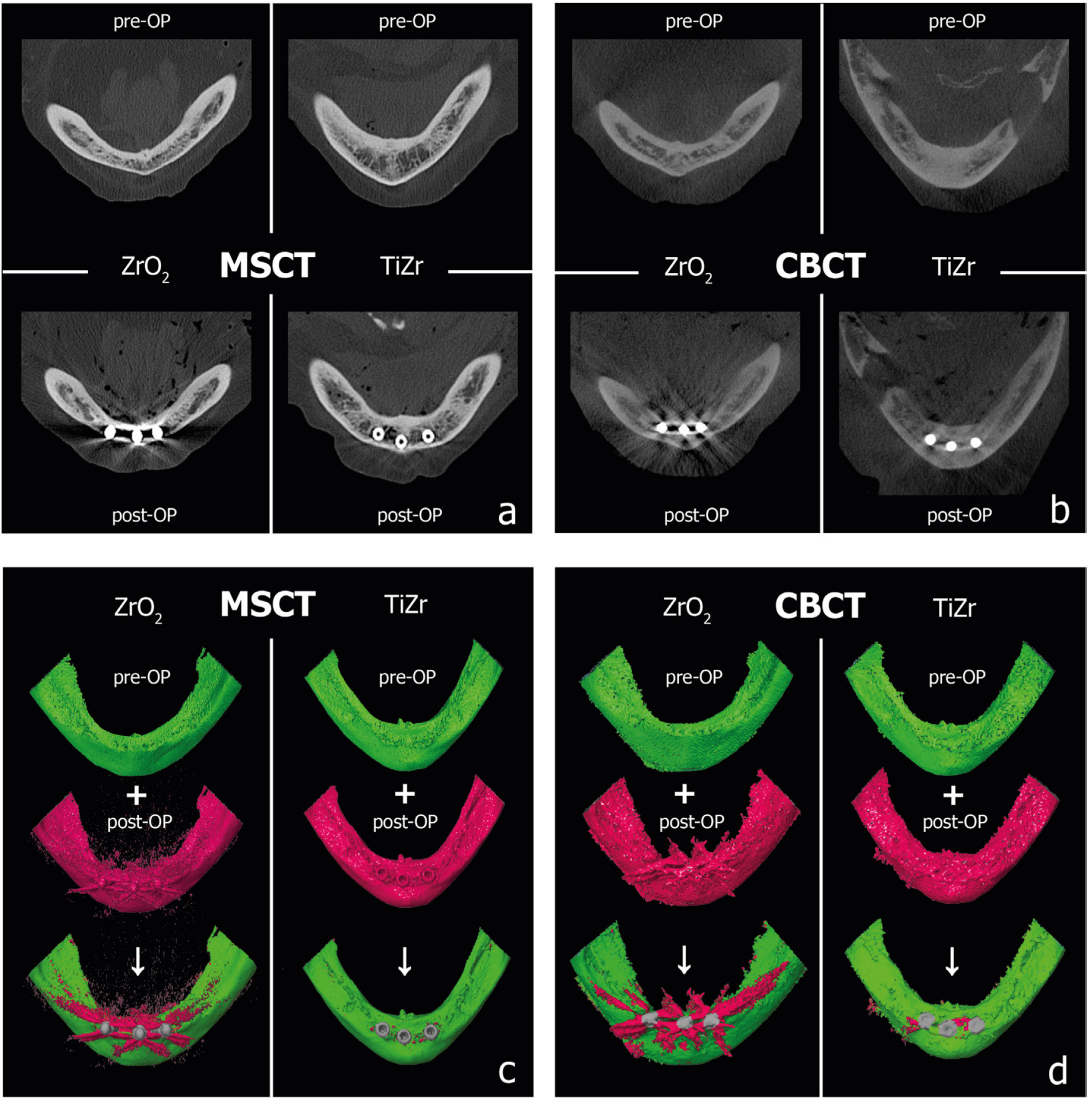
(a) Two-dimensional sectional view of the baseline and post-op scans with ZrO_2_ and Ti-Zr implants in the mandible in MSCT and (b) in CBCT. (c) Merging process of the corresponding STL models with 3D representation of the baseline models (green) and the artefact containing post-op models (red) for MSCT and (d) for CBCT. CBCT, cone beam CT; MSCT, multislice CT; STL, standard tessellation language.
